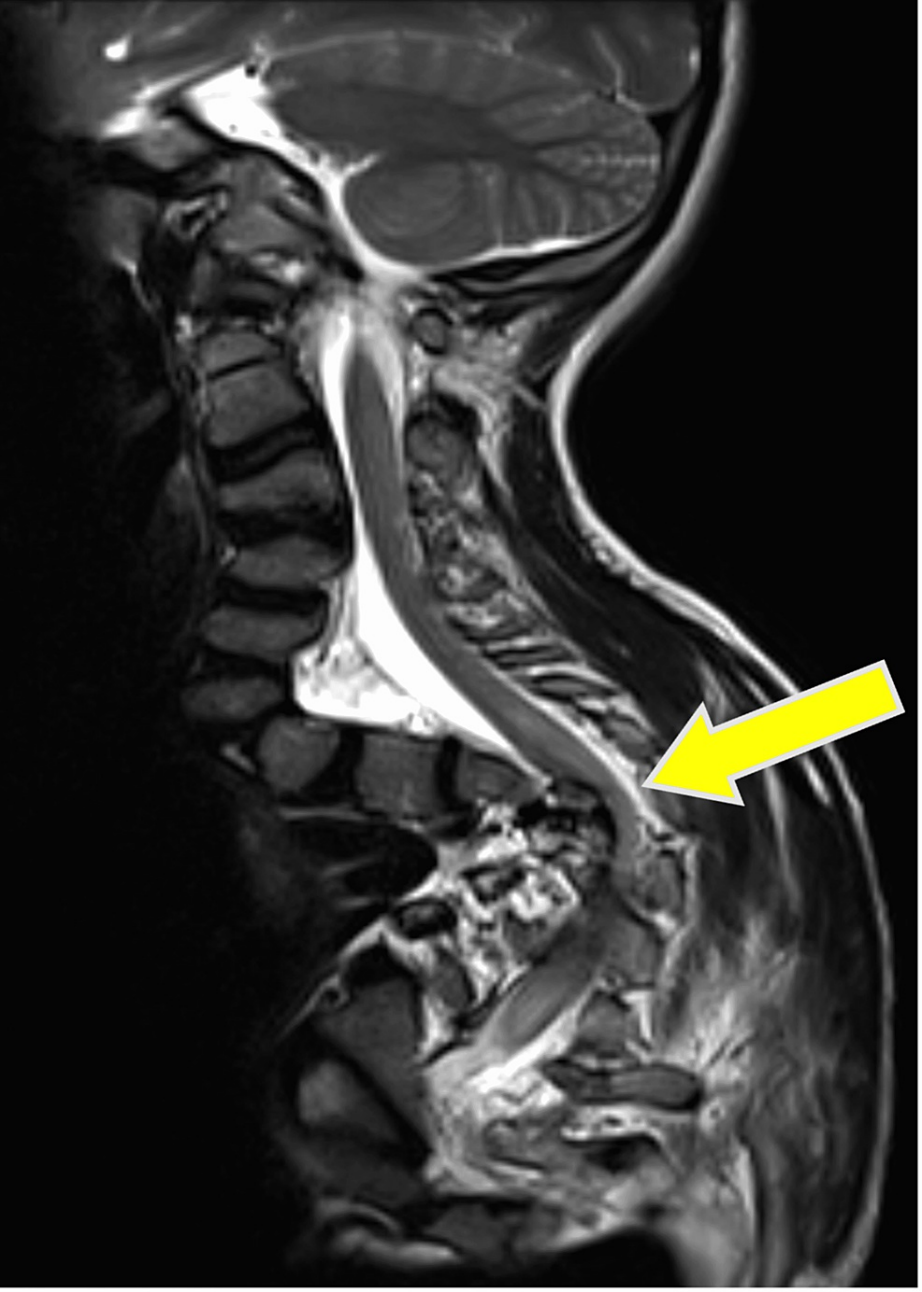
MRI showed high signal intensity within the spinal cord from the level T3 to T7
